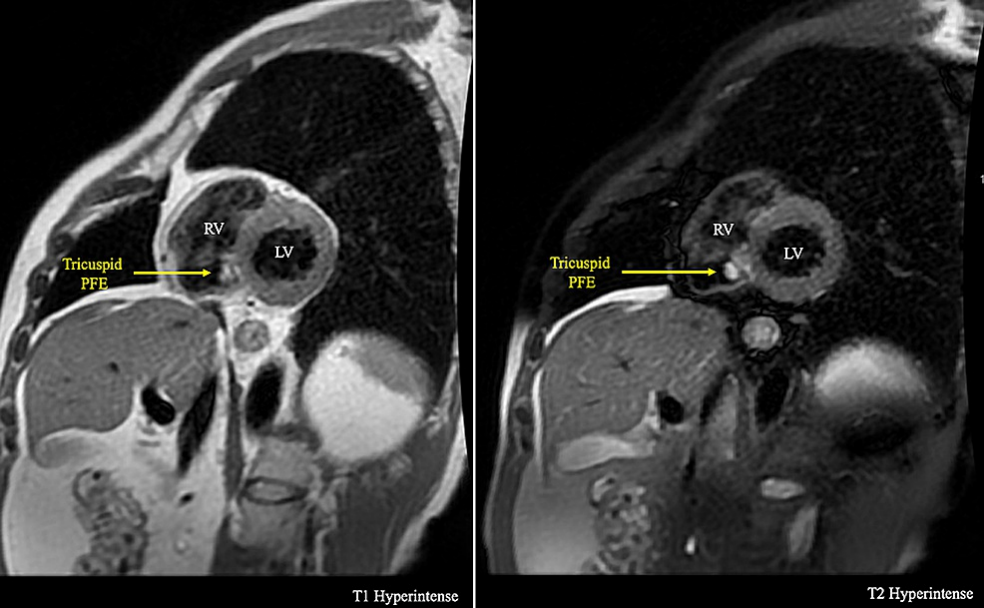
cMRI short-axis view showing T1 hyperintense (left) and T2 hyperintense (right) images depicting a cross-section showing a mass on the TV. cMRI, cardiac MRI; TV, tricuspid valve
